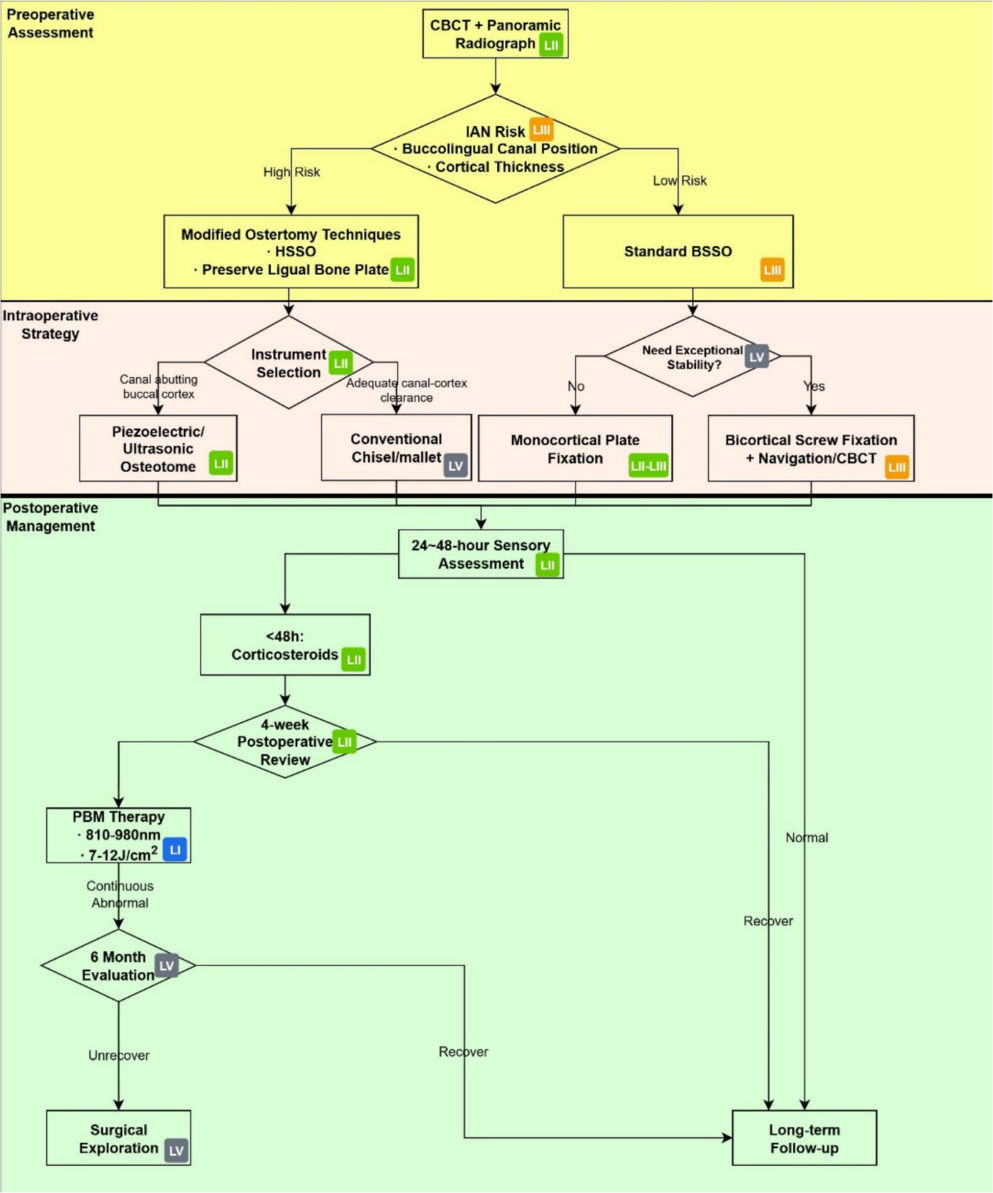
Multimodal clinical pathway for IAN injury prevention and management in BSSO. Abbreviations: BSSO, bilateral sagittal split osteotomy; CBCT, cone-beam computed tomography; HSSO, high oblique sagittal split osteotomy; IAN, inferior alveolar nerve; PBM, photobiomodulation. Evidence grading (OCEBM 2011): level I—systematic reviews/meta-analyses or high-quality randomized trials; level II—lower-quality randomized trials or prospective cohort studies; level III—retrospective cohort or case-control studies; level V—expert opinion/consensus. Ranges such as “level II–III” indicate mixed evidence
